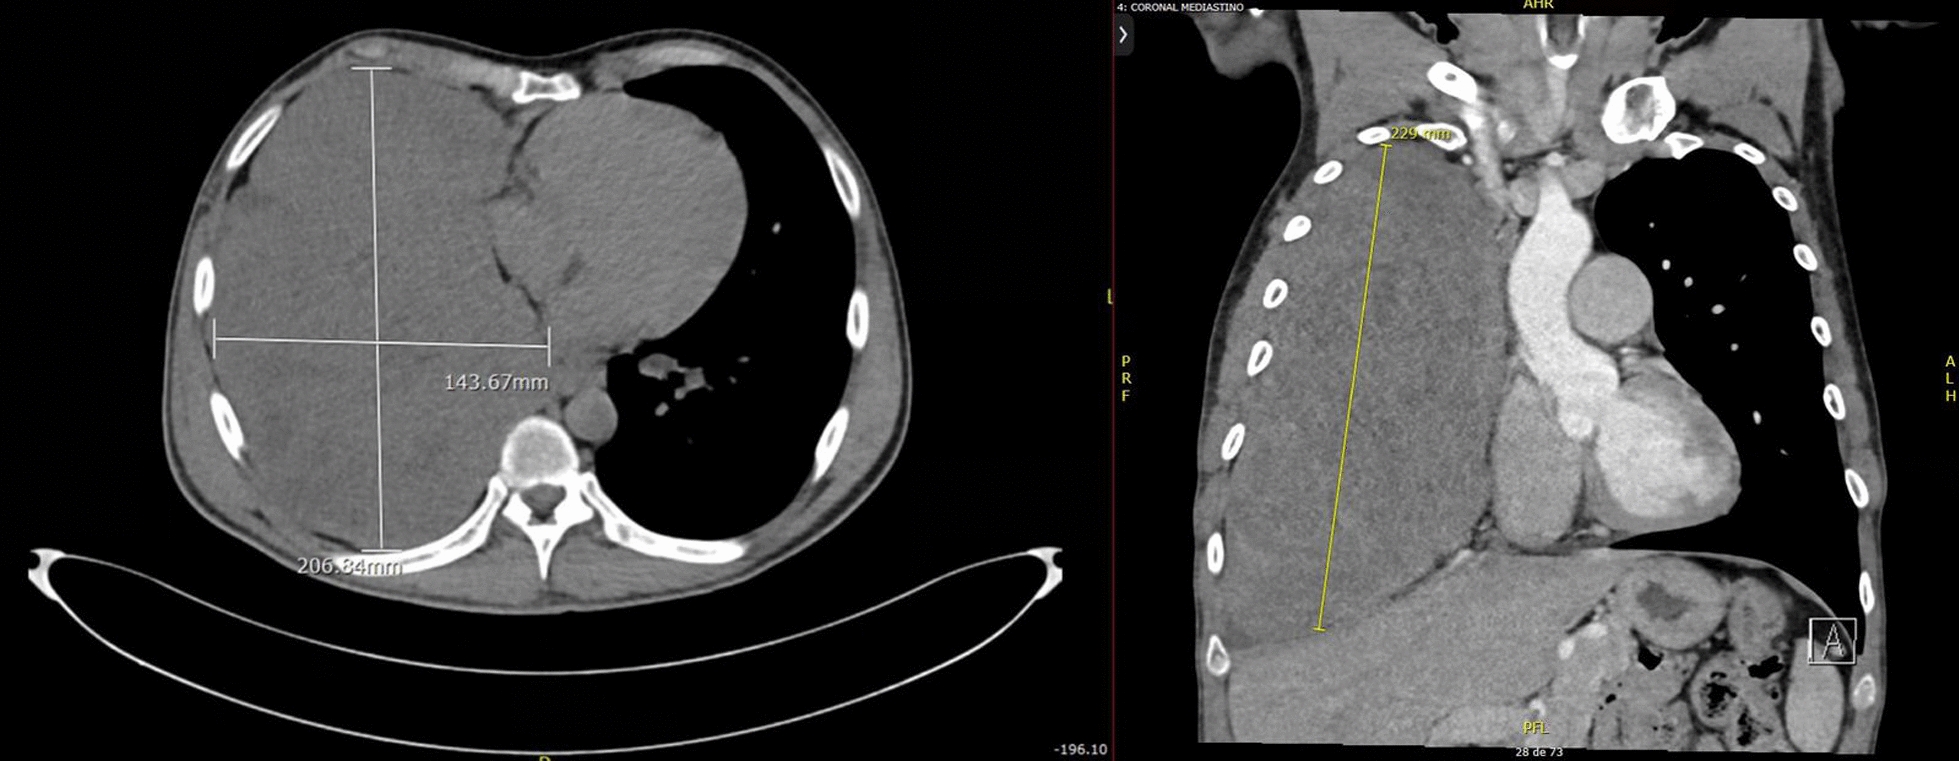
Chest computerized tomography (CT) of axial (left) and coronal (right) sections revealed a large solid mass with extrapulmonary features, demonstrating mild heterogeneous enhancement and some coarse calcifications in its lower portion, measuring approximately 229 by 204 by 124 mm, occupying nearly the entire right hemithorax. An apparent vascular supply was observed from branches of the right middle adrenal artery and right internal mammary artery
